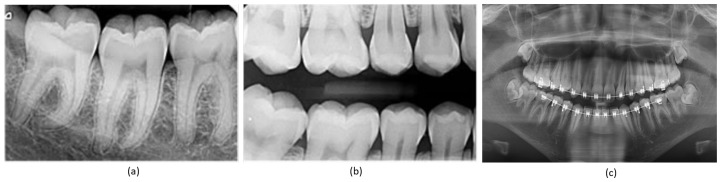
Types of X-ray images: (a) periapical X-ray; (b) bitewing X-ray; (c) panoramic X-ray.
